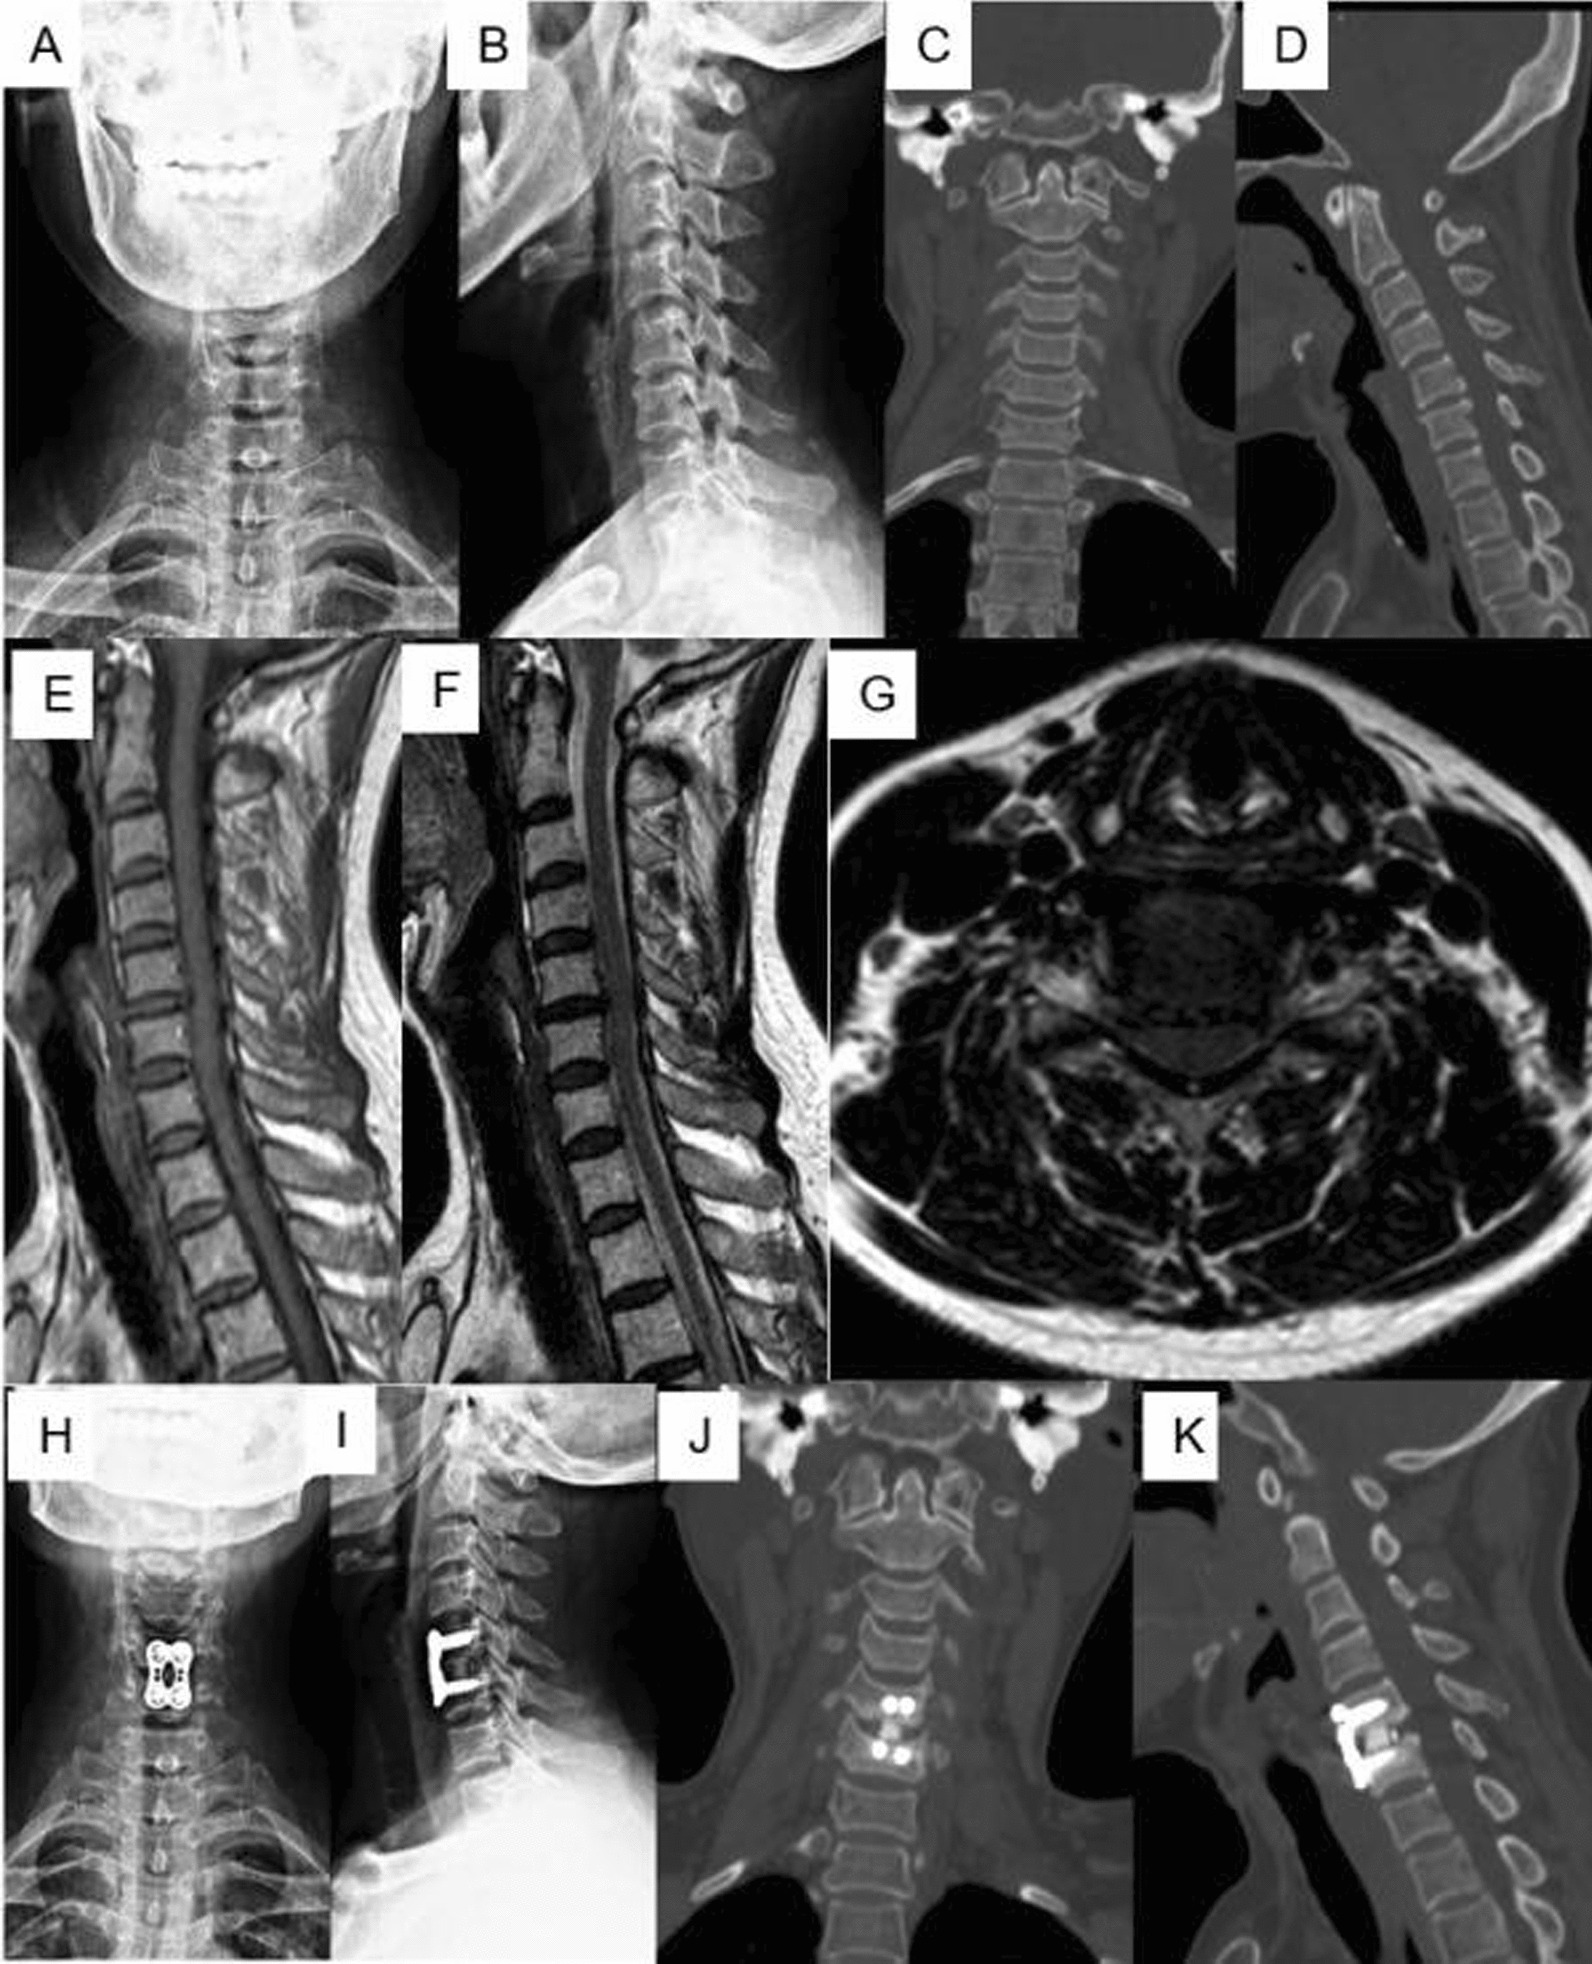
The patients underwent ACDF with plate and cage internal fixation system. A anteroposterior and B lateral of standing cervical X radiographs. C, D CT in 3D-sagittal reconstruction; E, F T1- weighted and T2-weighted MRI in sagittal plain, G MRI in axial plain of C5/6. Postoperative 6 months, H anteroposterior and I lateral of standing cervical X radiographs; J, K CT in 3D-sagittal reconstruction
